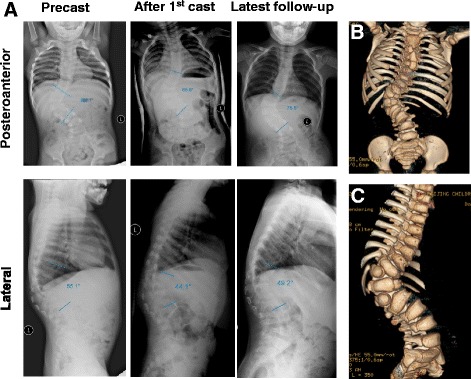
A representative case of CS patient (case no. 2). A 31-month-old female patient visited our clinic for back imbalance. X-ray radiographs revealed congenital scoliosis combined with kyphosis (a, *left panels*). Further CT examination revealed two hemivertebrae in thoracolumbar segment (b, c). The patient weighed 11.3 kg. After the first cast, both the degrees of scoliosis and kyphosis were reduced (a, *middle panels*). After four sessions of casting treatment during 13 months, the progress of scoliosis and kyphosis were both controlled (a, *right panels*). Patient weight increased to 14.2 kg at the latest follow-up
